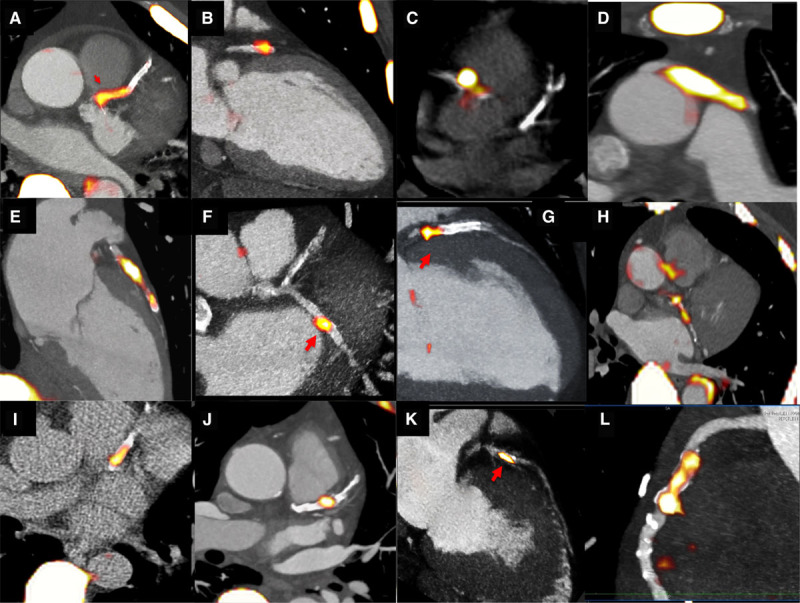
18F-fluoride PET imaging in the coronary arteries. Multiple examples of 18F-fluoride localizing to individual coronary plaques on fused positron emission tomography/computed tomography images of the heart. This can be observed in the left anterior descending artery (A, B, E, G, I, J, and K), circumflex (F and H) and right coronary arteries (C and L), and in a saphenous vein graft (D).
